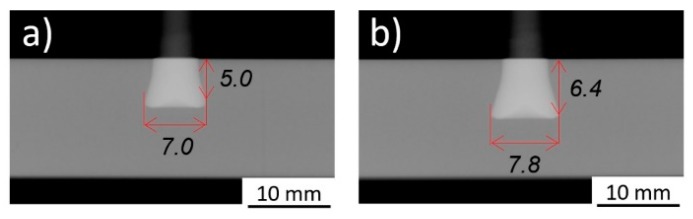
X-ray tomography of: (a) Condition 6 (RS = 20,000 rpm; FT = 1.6 s; FoT = 2 s; FF = 2000 N; FoF = 5100 N); (b) Condition 7 (RS = 18,000 rpm; FT = 2 s; FoT = 2 s; FF = 2000 N; FoF = 5100 N).
